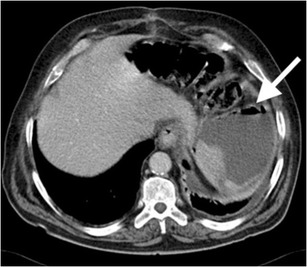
Splenic abscess. Axial contrast-enhanced CT of a 40-year-old woman demonstrates air (*arrow*) within a low attenuated splenic abscess. A culture test was positive for E.coli
